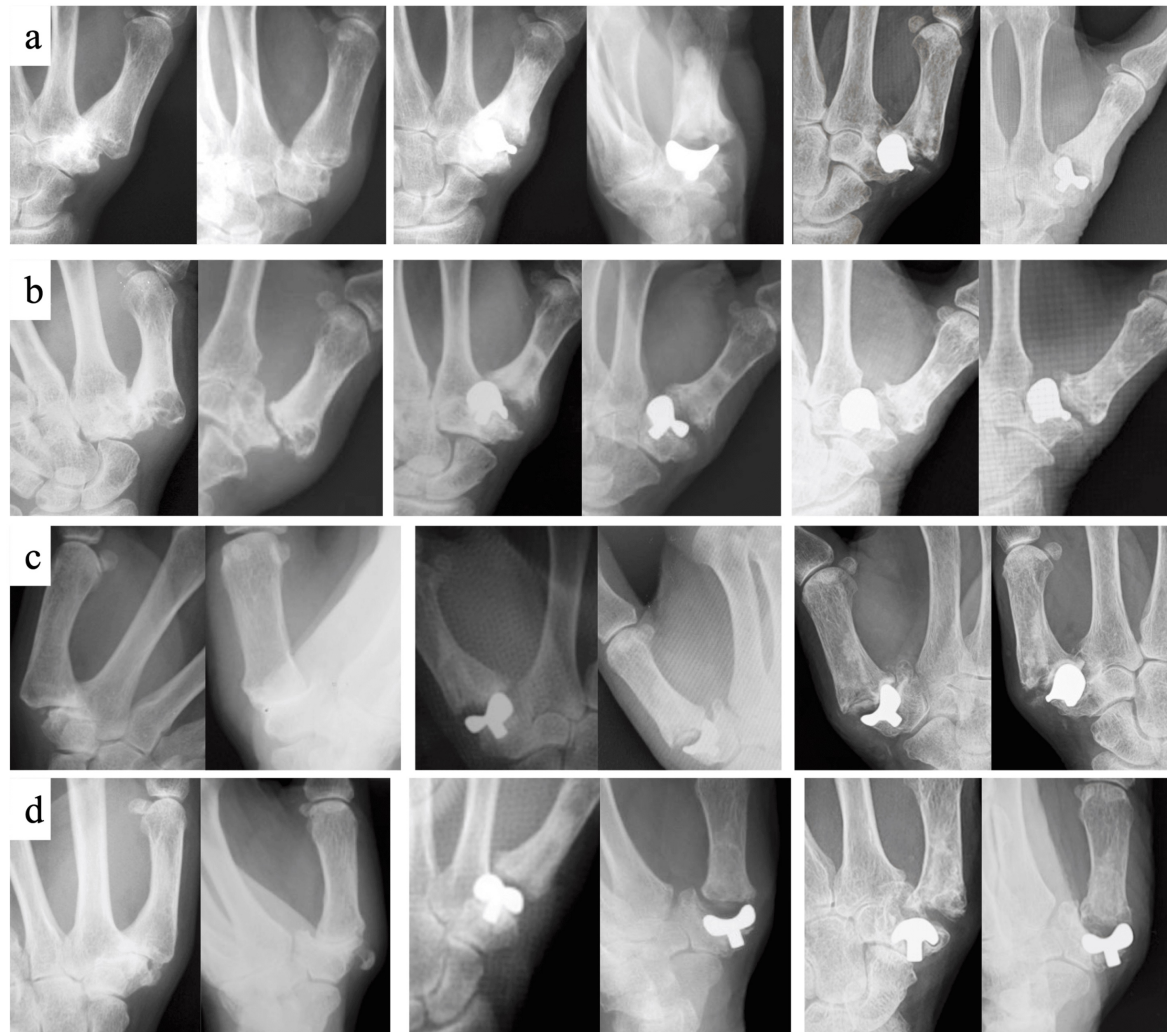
Postoperative radiographs (a: case 1, b: case 2, c: case 3, d: case 4): (left) preoperative, (middle) immediate postoperative, and (right) last observation (frontal and oblique views, respectively).
